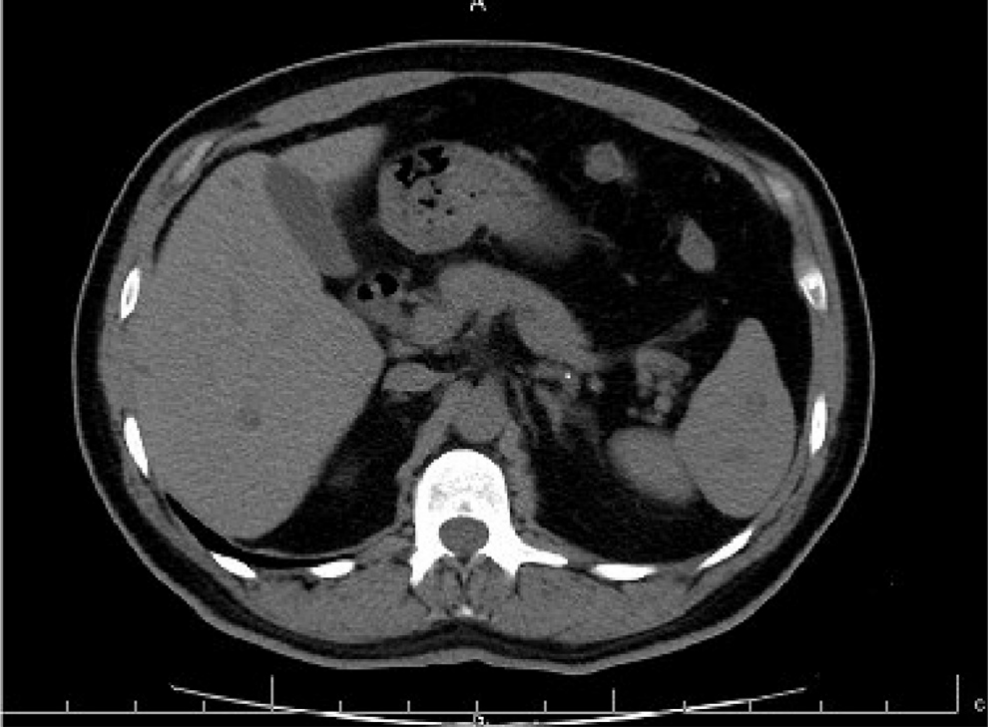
A round heterogeneous lesion which is hypodense in comparison with liver parenchyma, with density more than water measured up to 7 mm in the liver. It may contain internal echo or thin septations on ultrasound.
